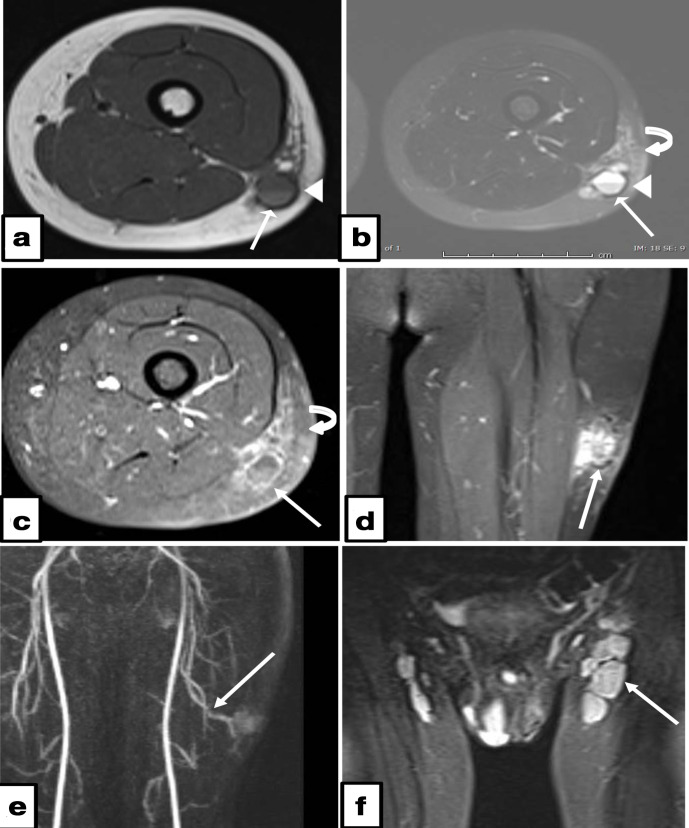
MRI of AFH. (a) Axial T1W and (B) axial T2 fat suppressed imaging shows a 3.4×2.3×2.6 cm heterogeneous ovoid subcutaneous lesion in the fat of the lateral aspect of the mid-left thigh without evidence of muscle infiltration (arrow). The lesion is isointense to muscle on T1WI with fluid-fluid level (arrowhead), and heterogeneously hyperintense on T2 fat saturation. The periphery of the mass demonstrates the typical low signal on non-enhanced images representing a fibrous pseudo capsule (arrows). (c) Axial T1 post gadolinium showing peripheral enhancement (blue arrow), with surrounding peritumoural soft tissue edema and enhancement noted (curved arrow in b & c). (d) Coronal T1 post-contrast imaging depicting variegated pattern of intralesional enhancement. (e) Static TWIST coronal MRA imaging of the left thigh with a prominent arterial branch from left profunda femoris artery noted to directly supply the tumour (yellow arrow). (f) Coronal STIR image of the inguinal regions with prominent ipsilateral inguinal lymph nodes (arrow).
